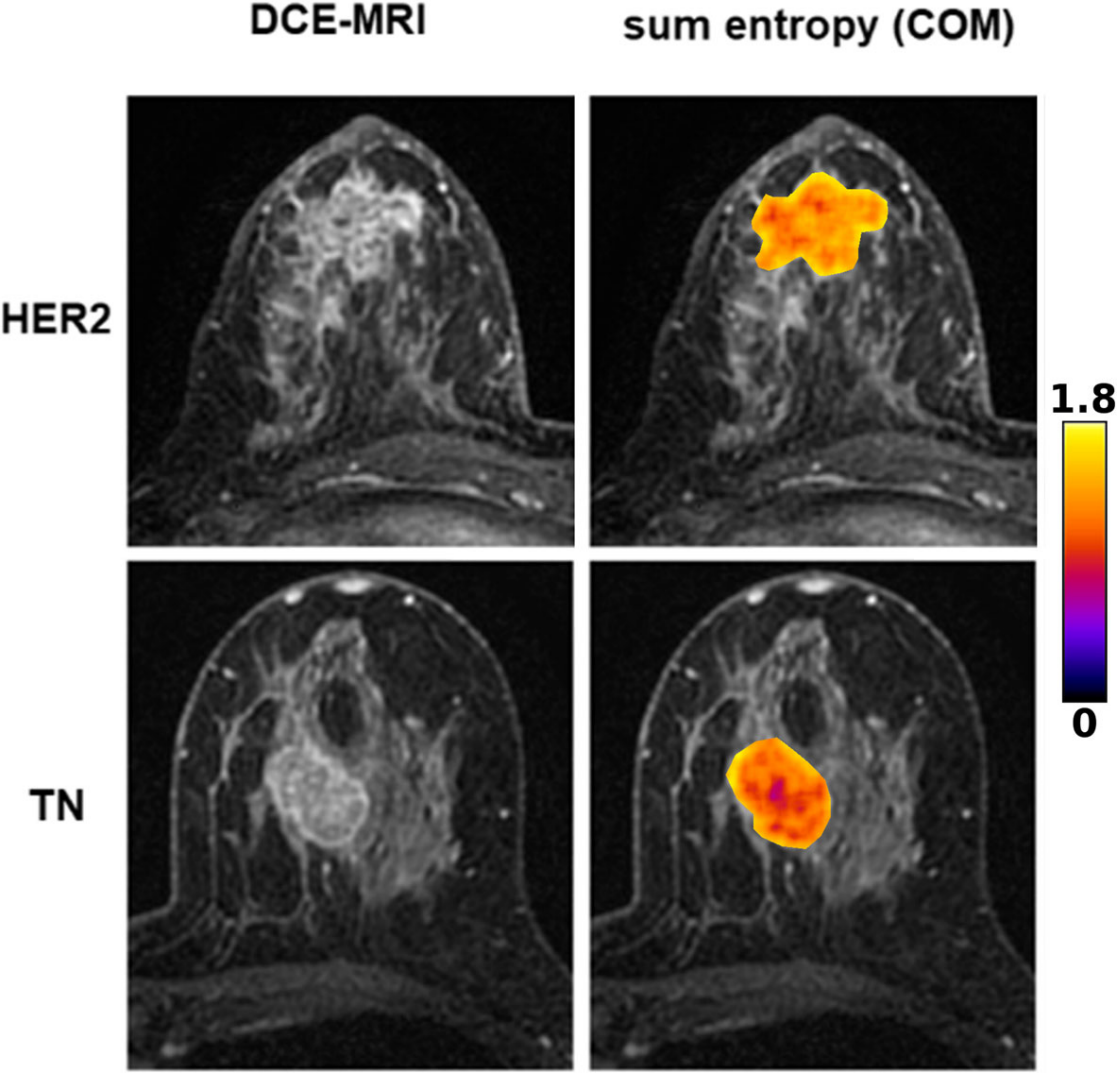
Original CE-MRI images and corresponding color-coded sum entropy feature map as overlay of the tumor area of triple-negative (TN) and HER2-enriched (HER2) breast cancer. TN shows a clearly lower sum entropy than HER2
